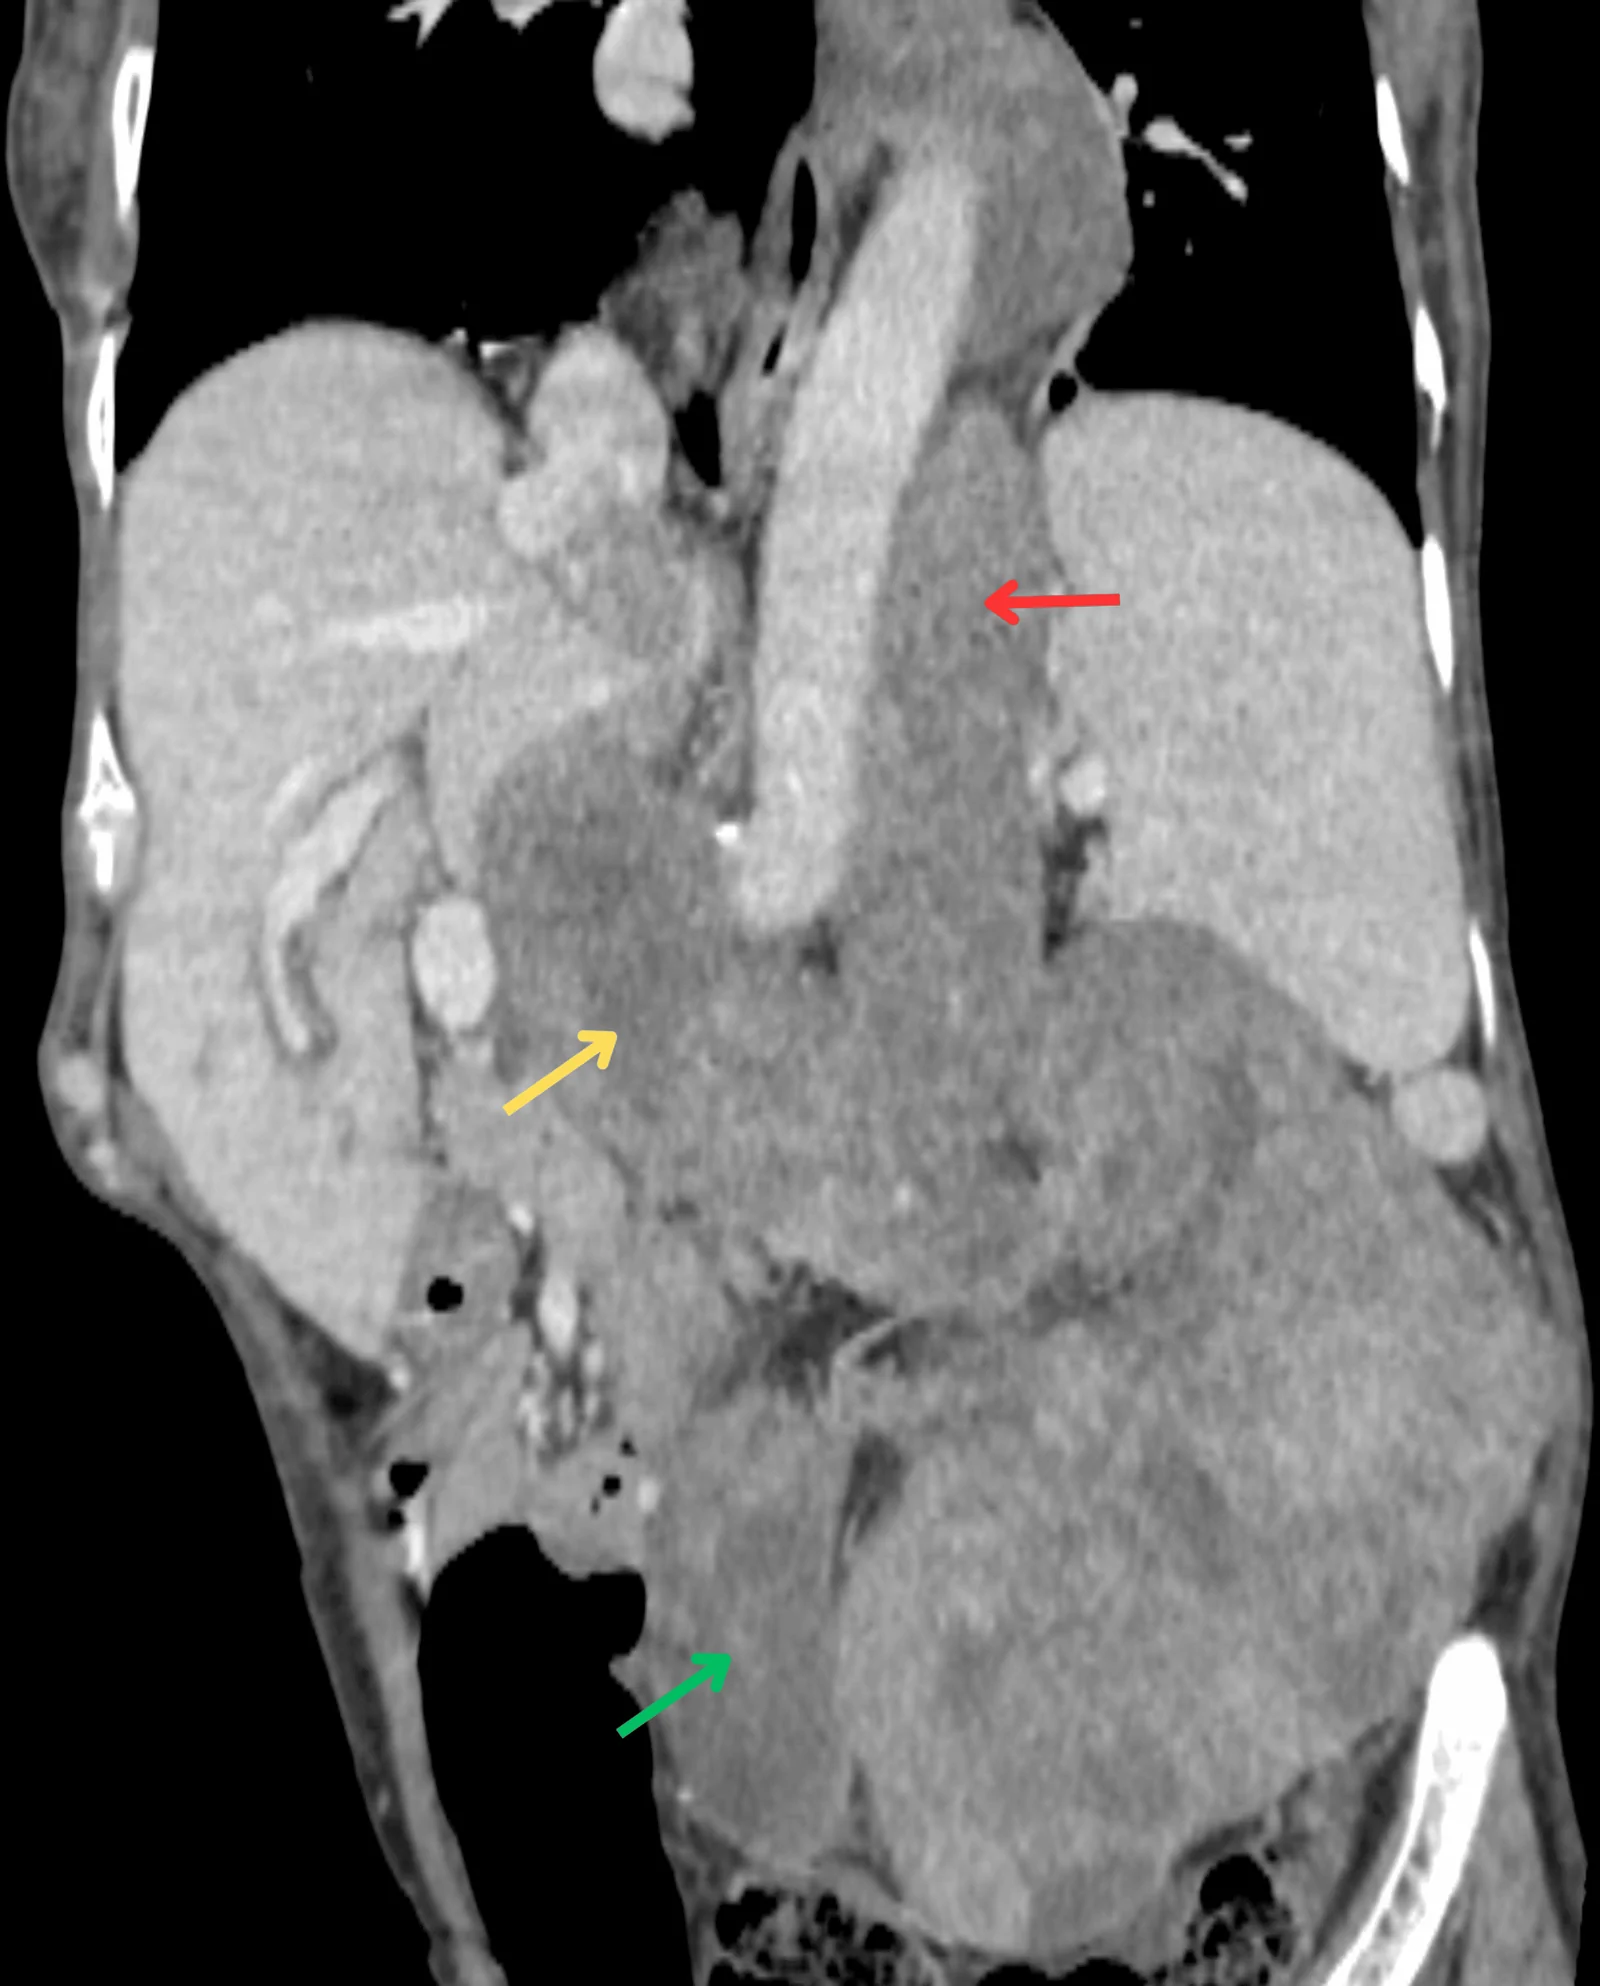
Coronal contrast-enhanced CT reconstruction demonstrating contiguous tumor thrombus extending from the left renal vein (yellow arrow) into the left gonadal vein (green arrow), with further cranial extension into the left ascending lumbar vein (red arrow).
